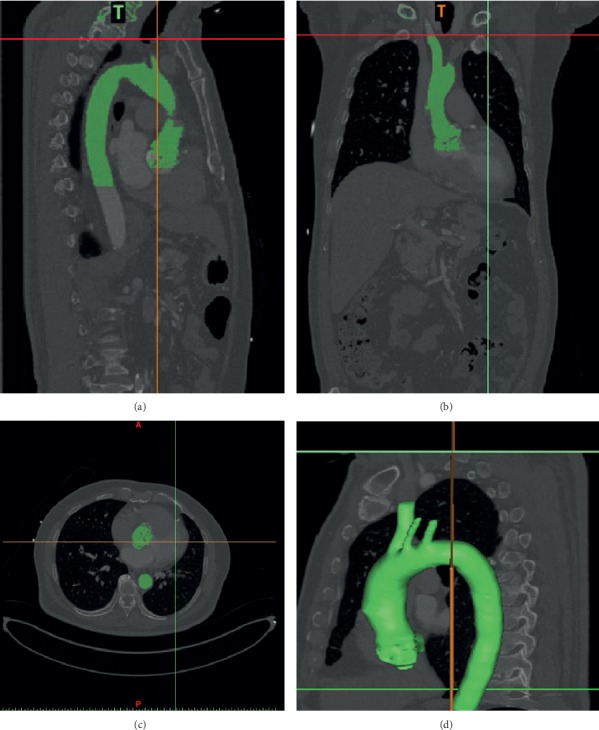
Aorta CT scan image from (a) sagittal (b) coronal (c) and axial planes. (d) 3D aorta model.
